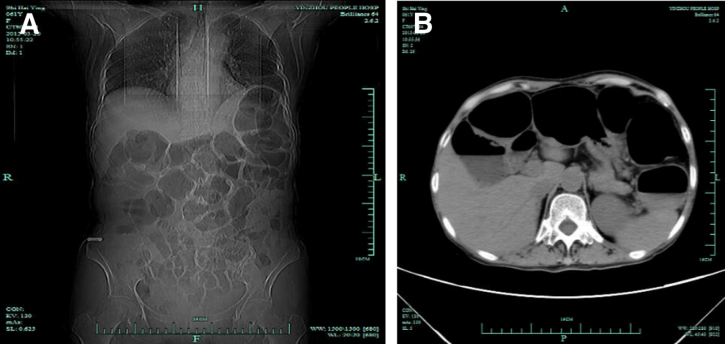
Plain radiography and abdominal CT image of the patient on January 4, 2014. Plain radiography shows marked intestinal fluid accumulation and pneumatosis intestinalis (A). Abdominal CT image reveals multiple air-fluid levels. (B) These findings indicate severe intestinal dysmotility and mucosal barrier disruption, consistent with chronic enteropathy secondary to Good syndrome. CT = computed tomography.
